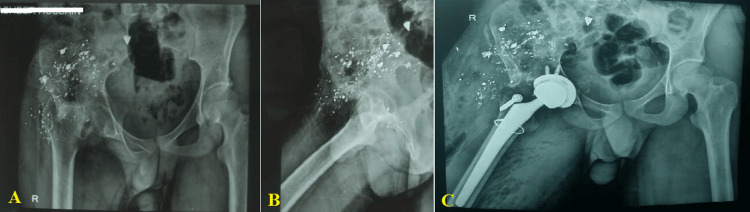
A radiologic X-ray shows (A) a 26-year-old male patient with a prior history of colostomy and contaminated hip joint after following a gunshot wound; anteroposterior view. (B) Fragment injuries within the right hip. (C) Seven months after the accident, the patient underwent right femoral shortening osteotomy and cement-free total hip arthroplasty; anteroposterior view. Image credits: Abdulrakib Almirah, author
